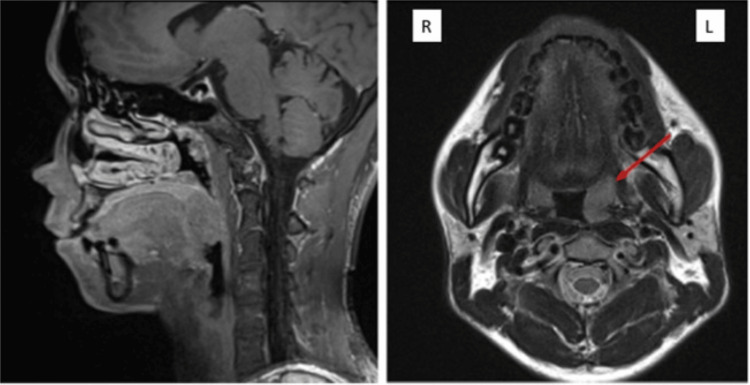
MRI assessment following treatment revealed complete resolution of the cervical lymph nodes and bilateral enlarged palatine tonsil lymph nodes, measuring AP 1.72 x LL 1.77 x CC 2.35 cm (red arrow) AP: Anteroposterior; LL: Laterolateral; CC: Craniocaudal.
